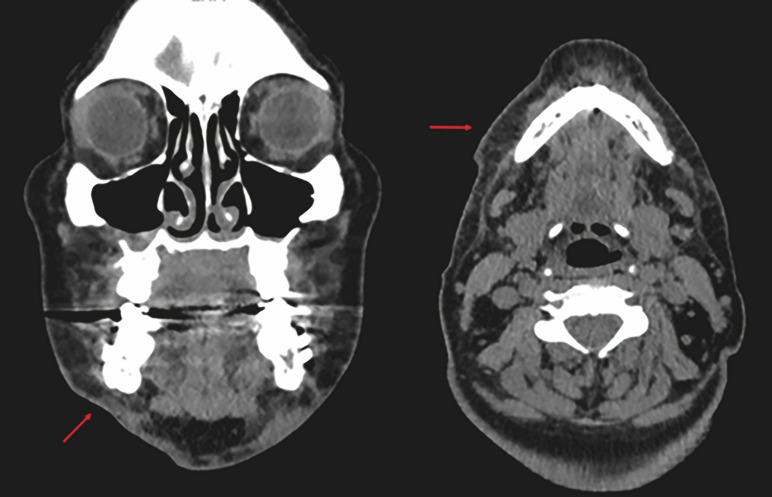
CT scan of the neck with contrast after emptying and surgical revision, coronal andaxial sections. Resolution of the infectious process without associated collections is visualized.
